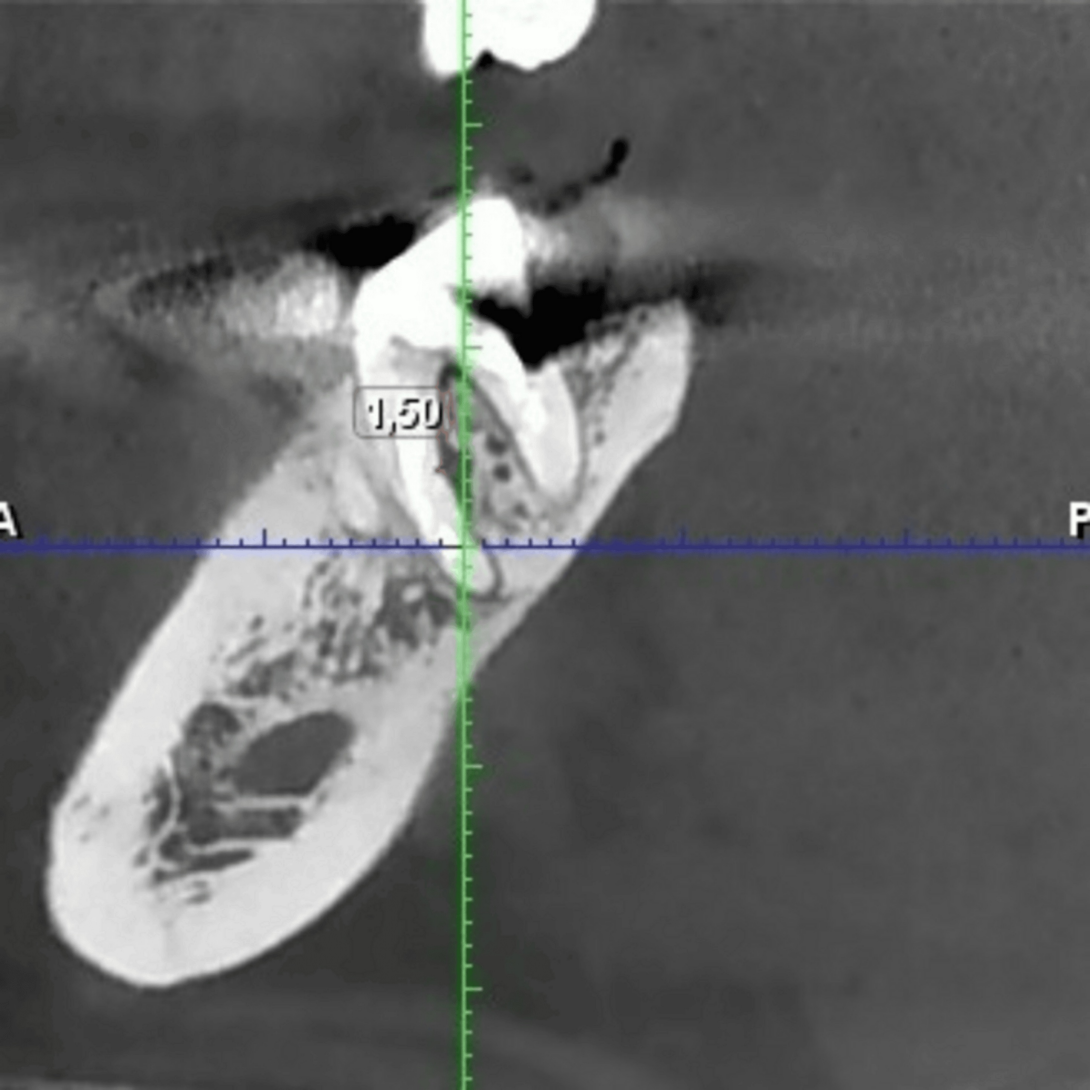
CBCT image of tooth 47 showing measurements of the interradicular defect, in sagittal section, after three months. CBCT, cone beam computed tomography.
